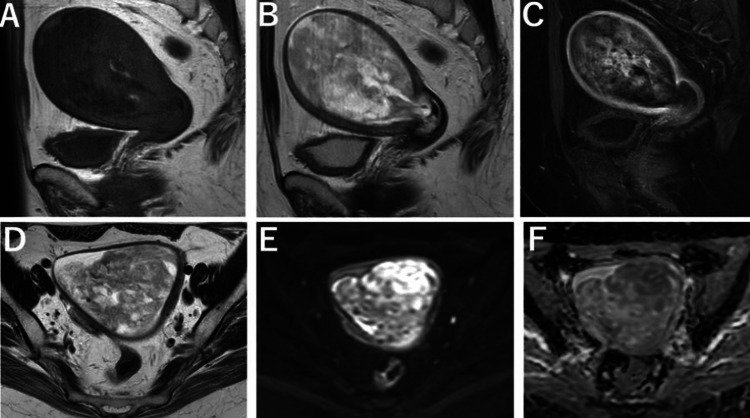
Findings on MRI. A) T1-weighted image (T1WI). B)T2-weighted image (T2WI): An 11×6 cm mass displaying mixed high signal intensity on T2-weighted images and isointense signal relative to the myometrium on T1-weighted images was observed, causing dilation of the internal cervical os.The mass contained cystic components. C) Fat-suppressed T1WI (40 seconds post-contrast) enhancement was observed in part of the tumor. D) T2WI. E) Diffusion-weighted image (DWI). F) Apparent diffusion coefficient (ADC) mild diffusion restriction was observed in part of the tumor.
